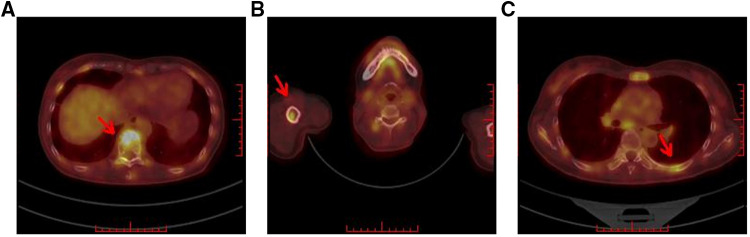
Breast molecular imaging findings from a 50-year-old woman with invasive ductal carcinoma with micropapillary features diagnosed with distant bone metastases. PET/CT images show the diffusely increased uptake of ^18^F-FDG in the following areas: (A) in the spine; (B) in the right humerus; and (C) in the left ribs (red arrows indicate the locations of metastatic lesions). Abbreviations: PET, positron emission tomography; CT, computed tomography; ^18^F-FDG, ^18^F-fluorodeoxyglucose radiotracer.
